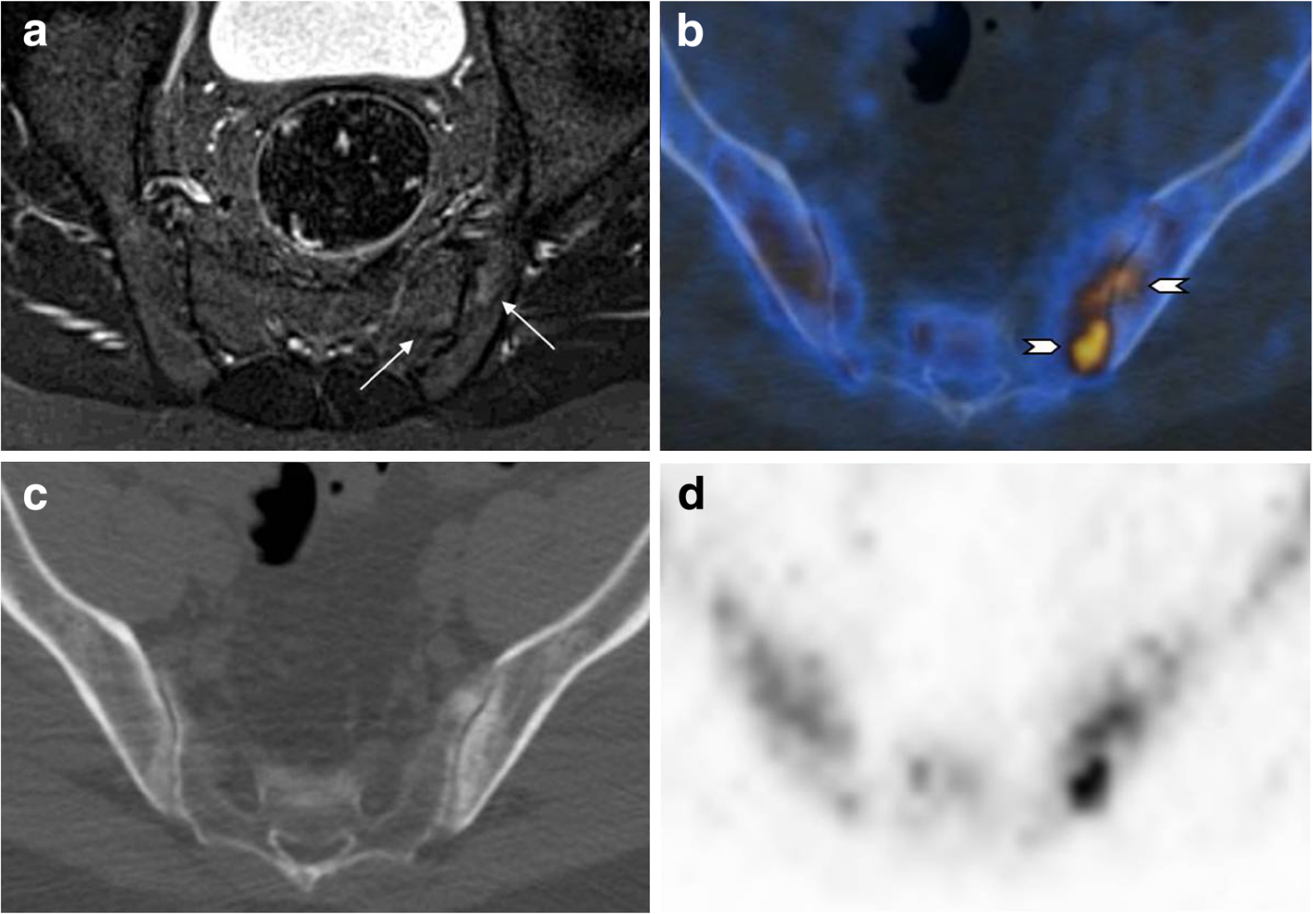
Thirty-two-year-old male patient (patient number10). Patient with positive findings in the inferior iliac and sacral quadrant of the left iliosacral joint both on MRI (edema, arrows) and on F PET/CT (arrowheads). Axial STIR (a), fused PET/CT (b), CT (c), and PET (d) images.
